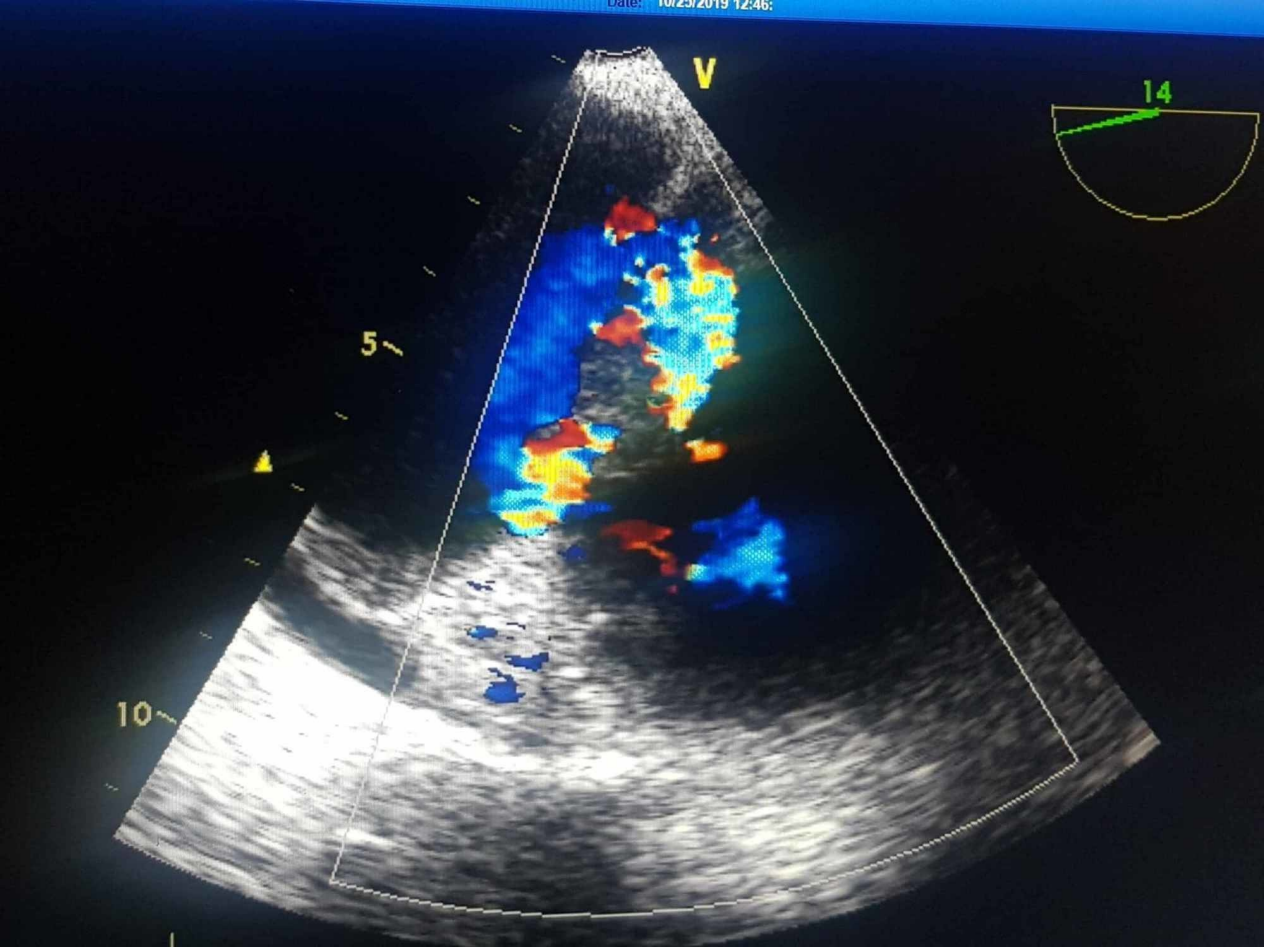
TEE revealing a large tricuspid valve vegetation and a perforated posterior tricuspid leaflet with moderate-to-severe tricuspid valve regurgitation. TEE, transesophageal echocardiography
